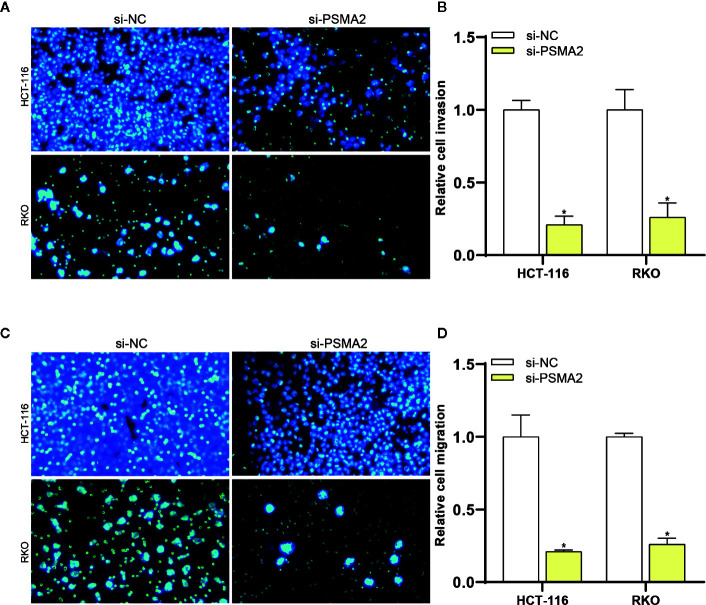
PSMA2 promotes CRC cell migration and invasion. (A, B) The migratory capacity of RKO or HCT-116 cells was inhibited after silencing PSMA2. (C, D) The invasive capacity of RKO or HCT-116 cells was inhibited by PSMA2 silencing. P* < 0.05.
